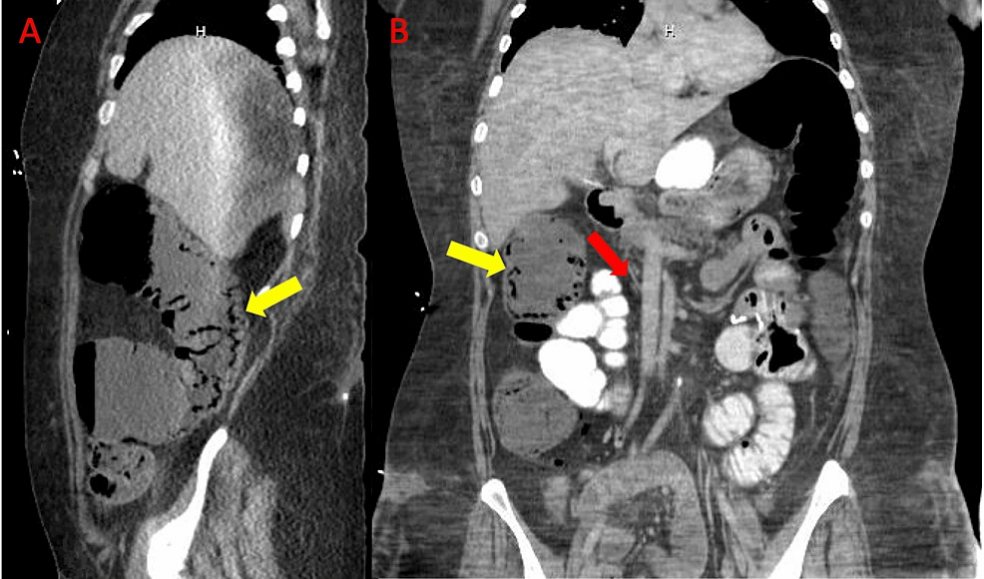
A: CT (sagittal view) redemonstrating pneumatosis coli of the ascending colon (yellow arrow). B: CT (coronal view) demonstrating gas in the mesenteric veins (red arrow). CT: computed tomography
